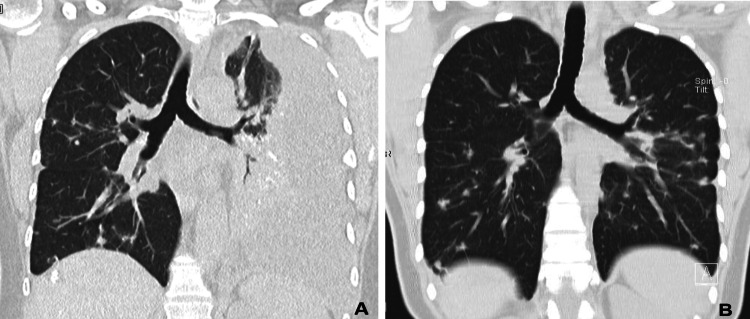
Coronal images of chest CT examinations at the carinal level at the time of current presentation to the emergency department (A) compared to a previous examination twenty years earlier (B) CT: computed tomography
